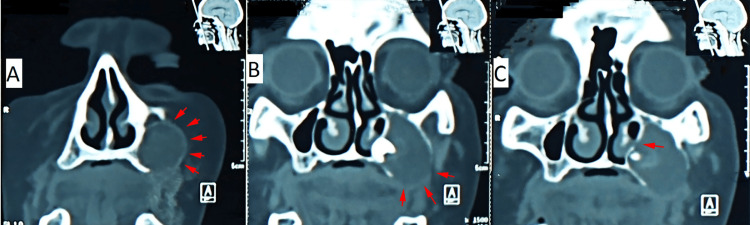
Multi-slice computed tomography (coronal sections). A: Large hypodense, expansile, well-demarcated, and osteolytic mass extending from the maxilla into the maxillary sinus (red arrows). B: An impacted third molar was abnormally displaced toward the lateral wall of the nose and the left inferior conchae with loss of the alveolar bone border and part of the lateral sinus wall (red arrows). C: Loss of the integrity of the medial wall of the sinus was noticed in a coronal section (red arrows).
